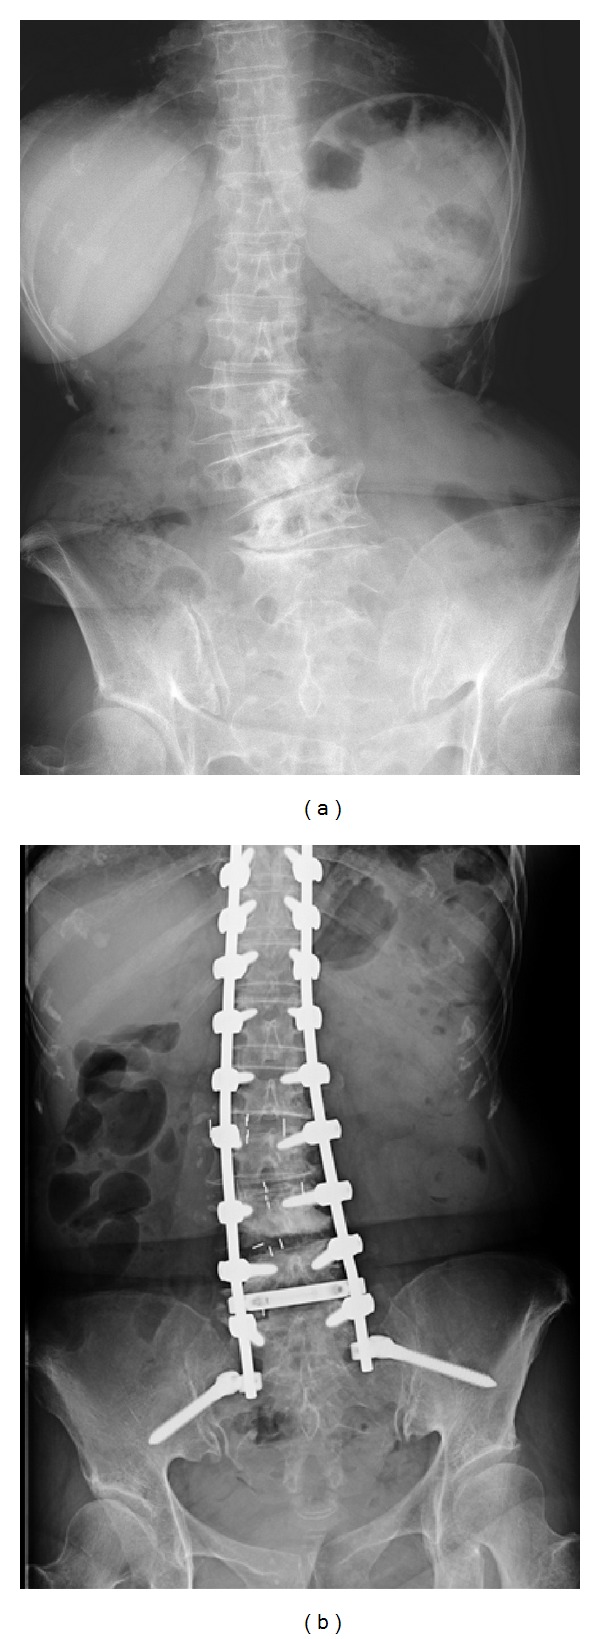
Magnification of X-ray film images of lumbar spine before and after surgery in Case 2. Anterior-posterior view (a) before surgery, and anterior-posterior view (b) after surgery.
